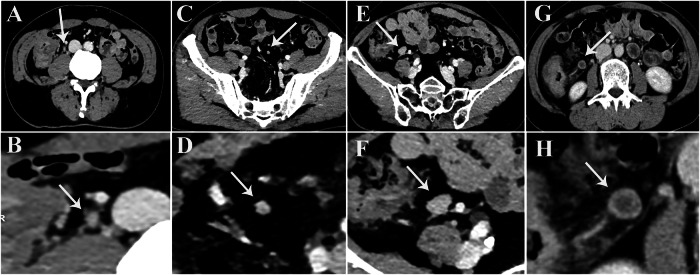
Representative examples of Node-RADS classification. The white arrows indicate the target lymph node. The upper row shows CT images acquired at a standard field of view, while the lower row presents corresponding magnified views of the target lymph node. A, B Cecal cancer with pT2N0 in a 58-year-old male. All readers evaluated the lymph node as normal size, homogeneous texture, smooth border, and kidney-bean-like shape, corresponding to Node-RADS category 1. C, D Sigmoid colon cancer with pT4N1 in a 63-year-old male. Evaluation in each reader was as follows: normal size (*n* = 6), heterogeneous texture (*n* = 3), smooth border (*n* = 5), spherical shape (*n* = 3). Node-RADS categories 1–2 were given by four readers, and categories 3–4 were given by two readers. E, F Ascending colon cancer with pT3N0 in a 73-year old female. Evaluation in each reader was as follows: enlarged (*n* = 6), homogeneous texture (*n* = 6), smooth border (*n* = 4), oval shape (*n* = 5). Node-RADS category 2 was given by four readers, and categories 3–4 were given by two readers. G, H Ascending colon cancer with pT3N1 in a 59-year-old female. All readers evaluated the lymph node as enlarged, gross necrosis, smooth border, and spherical shape, corresponding to the Node-RADS category 5
